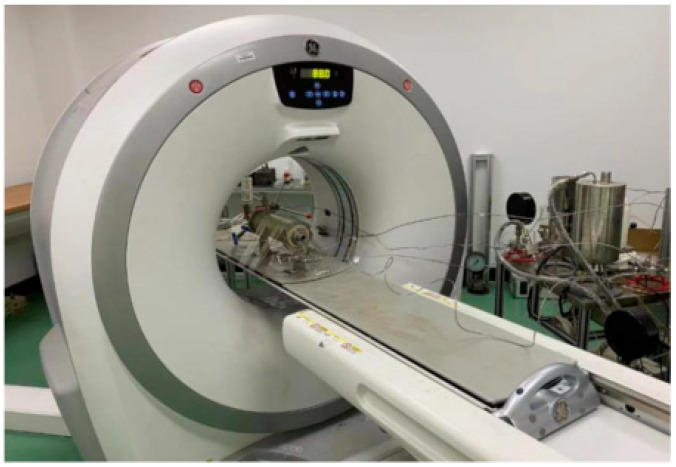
Micron precision CT scanner.
